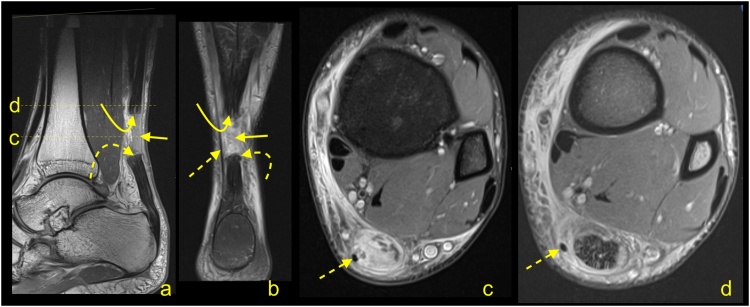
Achilles tendon rupture in midportion (straight arrow). A 36-year-old patient receiving systemic corticosteroid therapy. MRI (a–d) revealed that no adhesions are visible between the proximal stump (curved arrow) and distal stump (dashed curved arrow). Very regular outlines of stumps are visible. The plantaris tendon is preserved (dashed arrow). A- PD- weighted, b- T2- weighted with fat suppression, c and d- PD- weighted with fat suppression.
